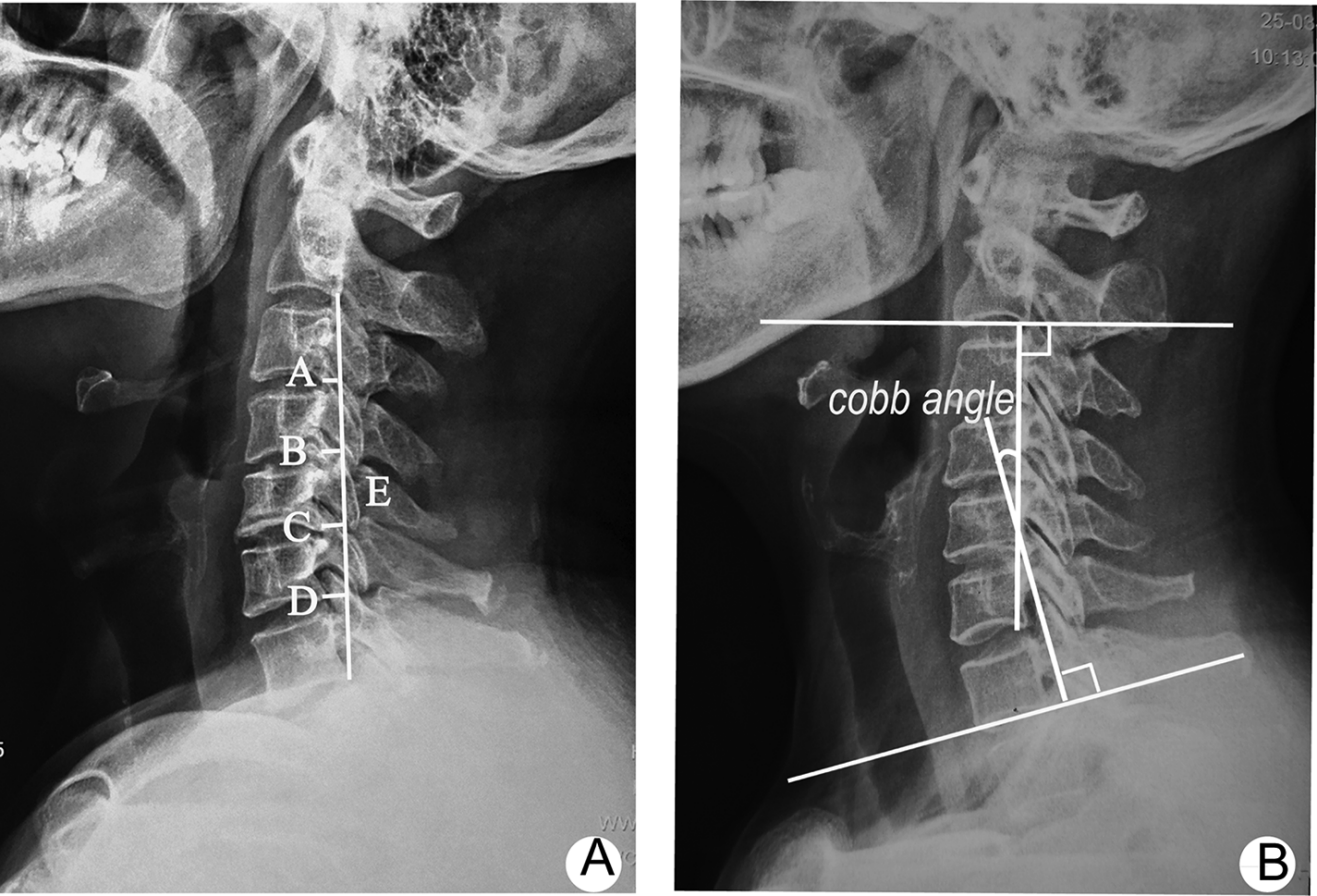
Radiological parameters of the cervical spine. (A) CCI measures cervical curvature based on the distance from the posteroinferior edge of the C3–C6 vertebral body to a straight line drawn from the posteroinferior edge of C2–C7 [CI = (A + B + C + D)/E × 100]; (B) Cobb angle measures the cervical lordotic angle formed by two lines perpendicular to inferior endplates of the C2 and C7 vertebral bodies, respectively. Cervical range of motion (ROM) was calculated as the difference between the Cobb angles at maximal flexion and extension on anteroposterior (AP) radiographs.
